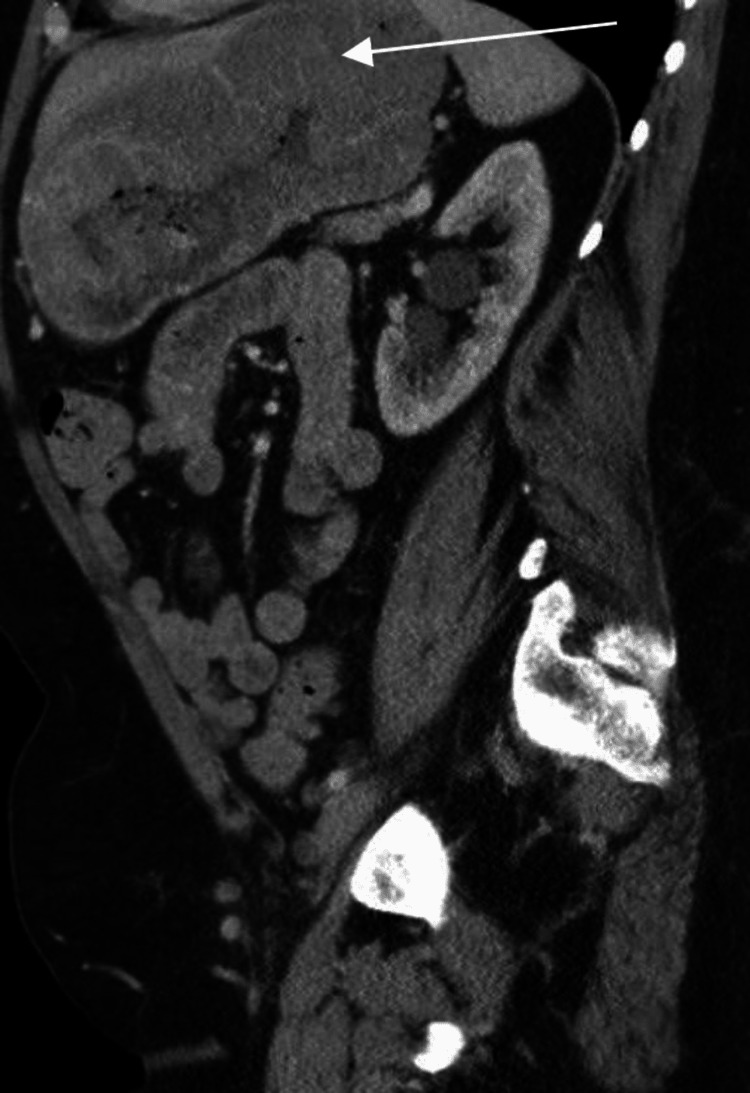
Sagittal IV contrast-enhanced CT image demonstrates marked gastric wall hypertrophy (white arrow)
